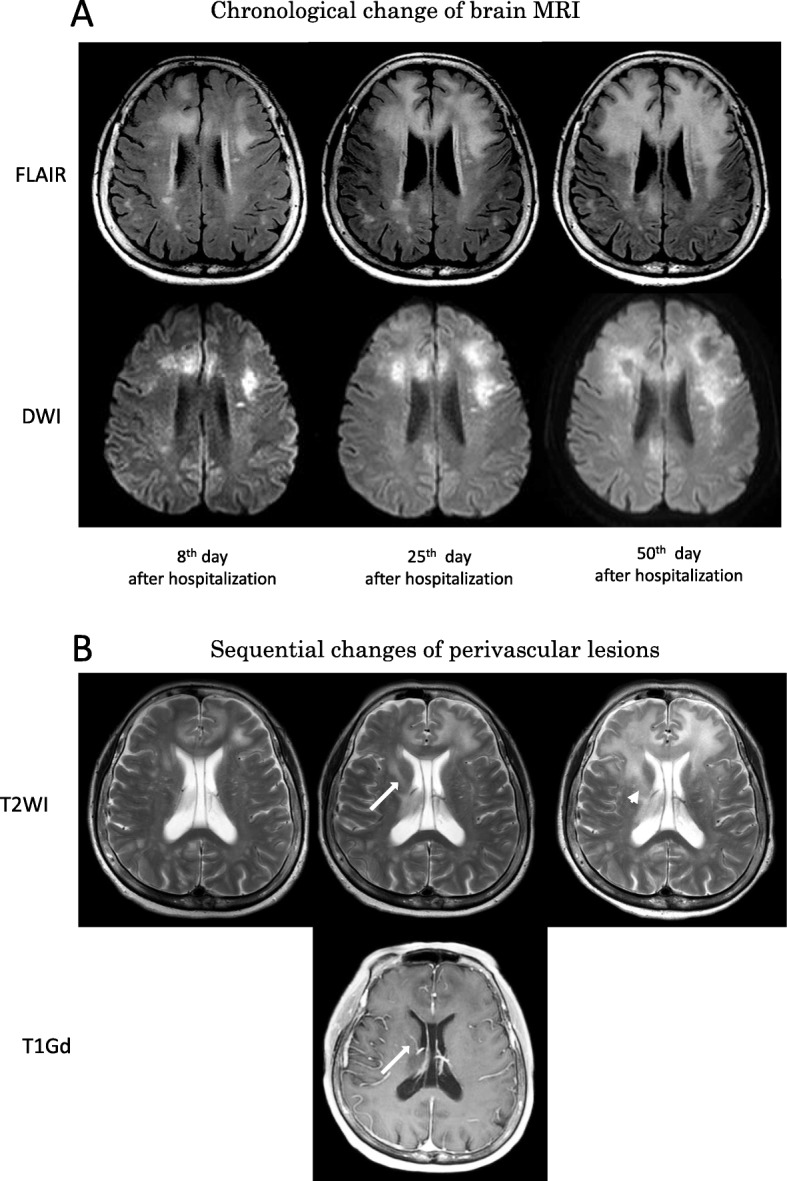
Imaging findings of this patient. a Chronological changes in brain magnetic resonance imaging (MRI), fluid attenuated inversion recovery (FLAIR; top row), and diffusion weighted imaging (DWI, bottom row) on an axial view. Eight days following the patient’s admission, a FLAIR image revealed a high signal lesion in the cerebral white matter, which was dispersed in the central bilateral frontal lobes. Signal elevations were also revealed on DWI. (left) Twenty-five days following the patient’s admission, the number of high signal white matter lesions increased on FLAIR and DWI, and the discrete lesions expanded. (middle) Fifty days following the patient’s admission, the lesion with an elevated FLAIR signal expanded further to cover the entire bilateral frontal lobes. On DWI, the patient’s lesions had altered signal facilitation in the center and a restricted signal along the edge with perilesional edema. (right). b T2-weighted magnetic resonance (MR) images (top row) demonstrated signal changes in the perivascular lesion. T1-weighted magnetic resonance imaging with contrast administration 25 days following the patient’s admission revealed enhanced vasculature (likely veins). (white arrow) Subsequently, a T2-weighted image taken 50 days after the patient’s admission revealed perivascular edema around the indicated blood vessel. (white arrowhead) Similar perivascular edema was identified in nearby blood vessels.
